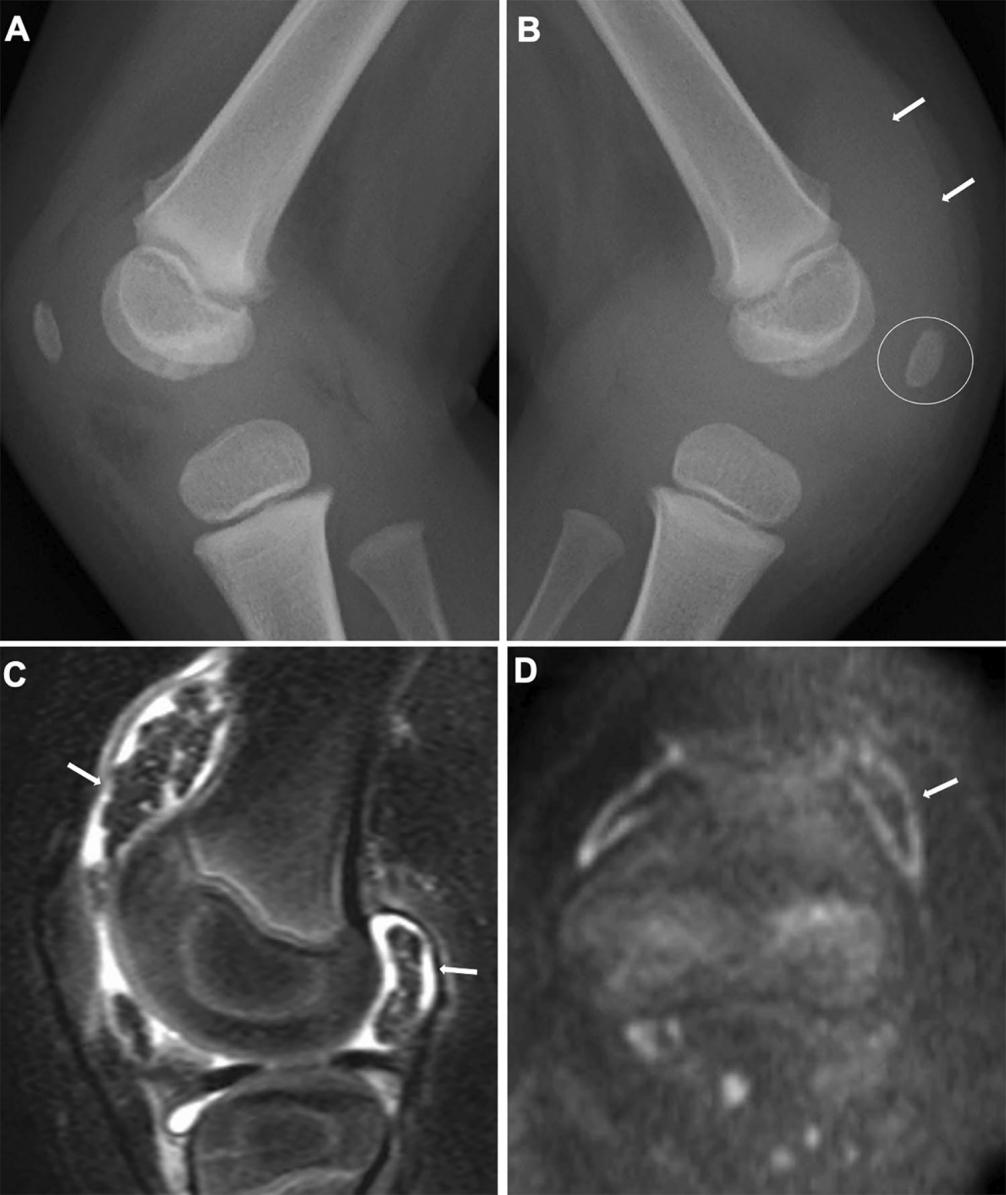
Oligoarticular type JIA. A-D. A 5-year-old girl with left knee pain. The lateral radiographs of the knees showed distension of the suprapatellar bursa (arrows, B) and accelerated patellar ossification (circle, B) of the left knee and a normal finding of the right knee (A). In the left knee, sagittal fat-suppressed T2-weighted sagittal image showed low signal rice bodies (arrows, C), and axial DWI demonstrated high signal intensities of the synovium, suggesting active synovitis (arrow, D)
